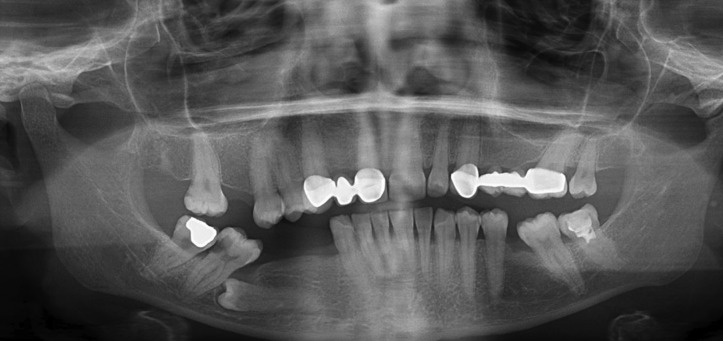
Extraoral panoramic radiography of the patient.
